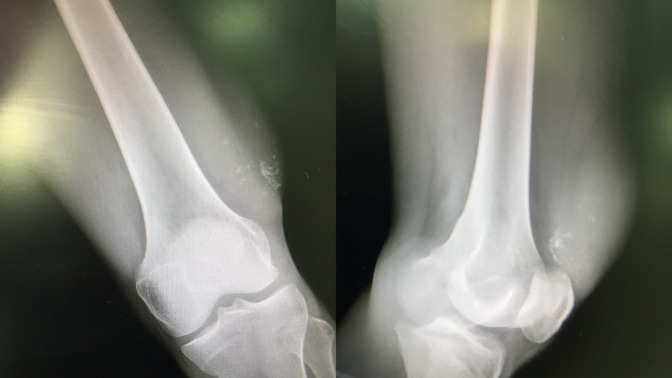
AP and oblique radiographs of the distal femur showing a soft-tissue mass near the knee joint with calcifications. These characteristic findings lead to synovial sarcoma being in the differential of possible lesions. The mass was mobile and away from the neurovascular structures and a chest radiograph was normal. Therefore, limb-salvage surgery could be considered.
